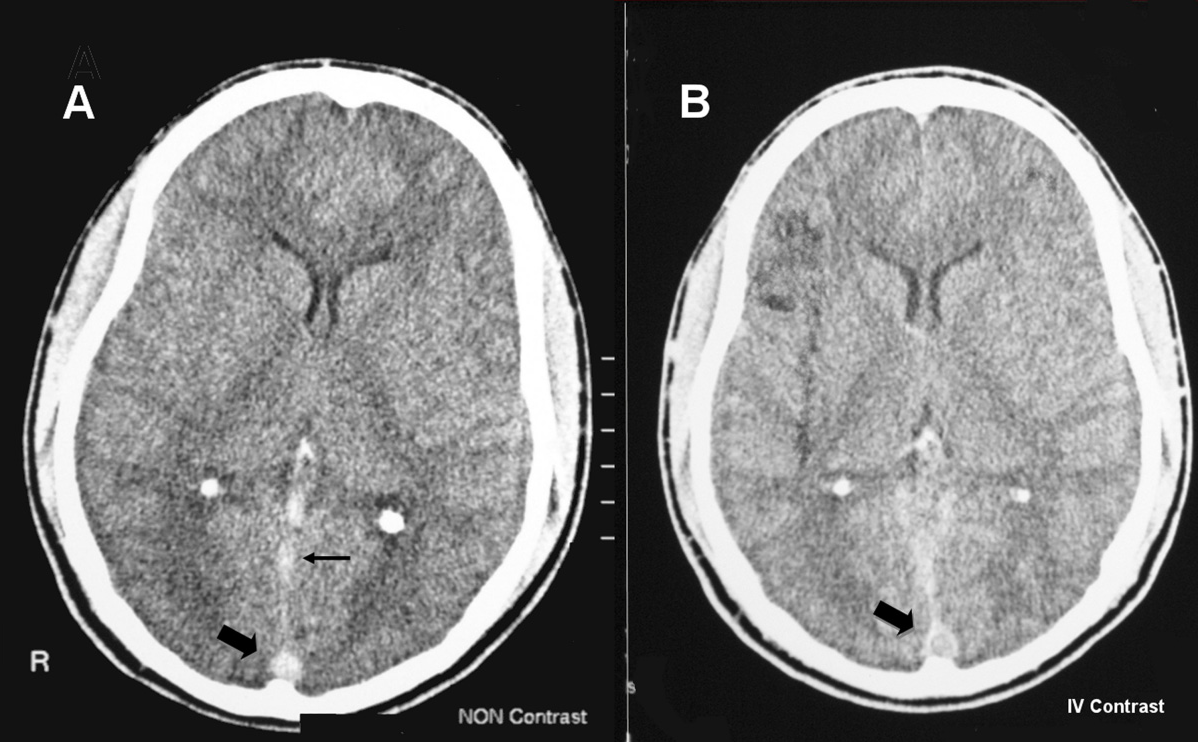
A. Non contrast enhanced CT scan showing the dense delta sign (thick arrow), with hyperintensity of the sagittal sinus (thin arrow). B. Contrast enhanced CT scan showing the empty delta sign (thick arrow).
